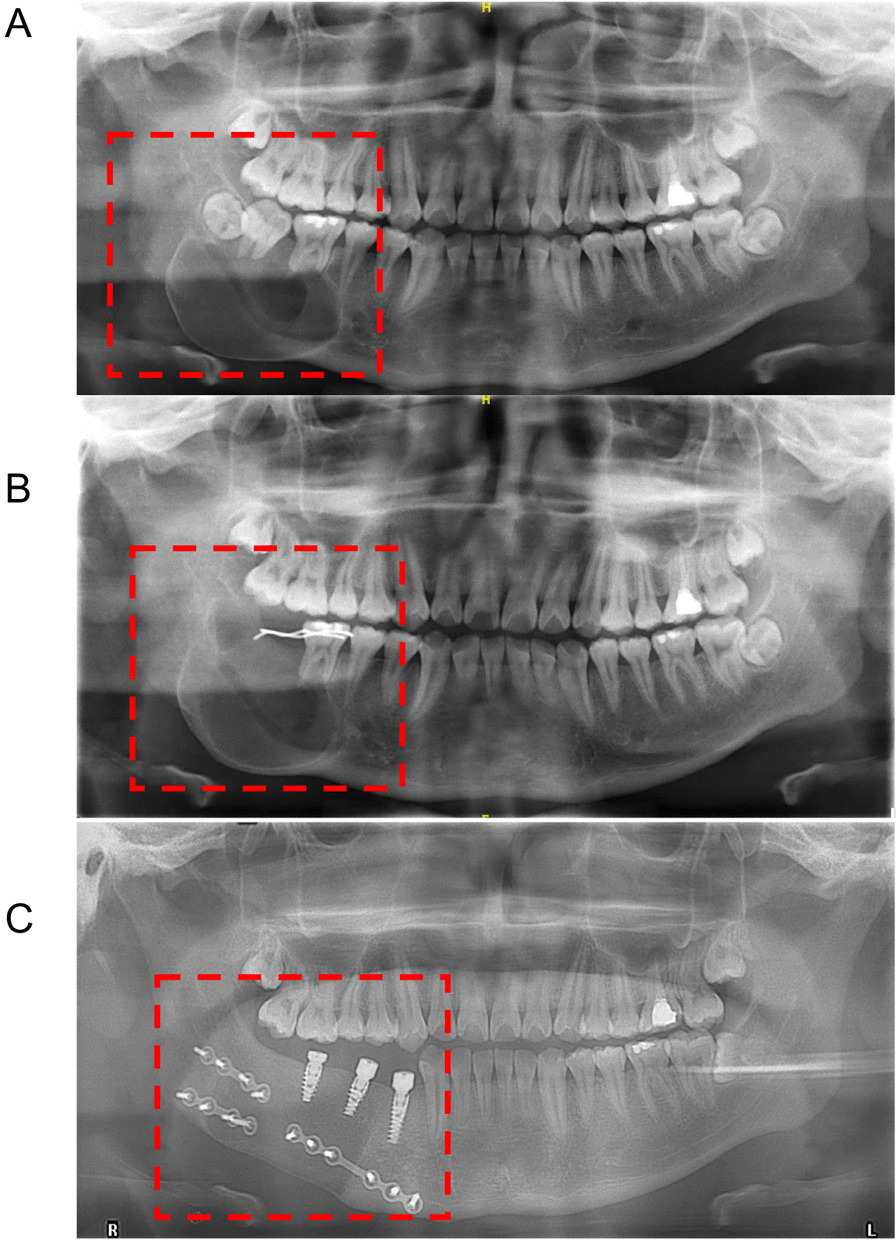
A case presentation of postoperative recurrence of UA. a Pre-operative panoramic radiography of patients; b Slight enlargement of the cystic cavity half a year after fenestration decompression; c A pantomography after the surgery of “Partial resection of right mandible + construction of vascularized free iliac myocutaneous flap”
